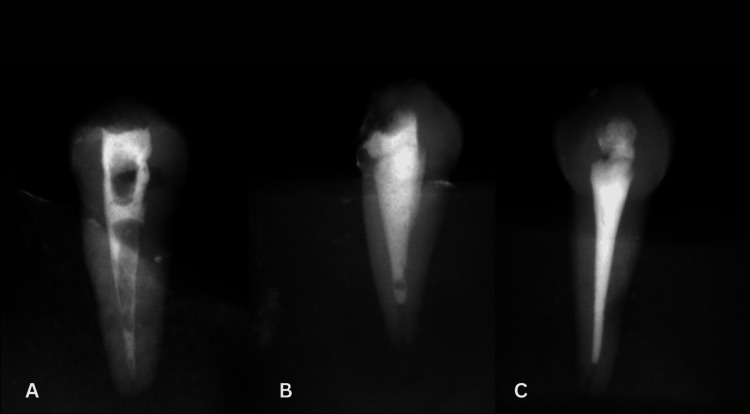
Radiographs showing the obturation density A.Unacceptable. B. Acceptable. C. Good
